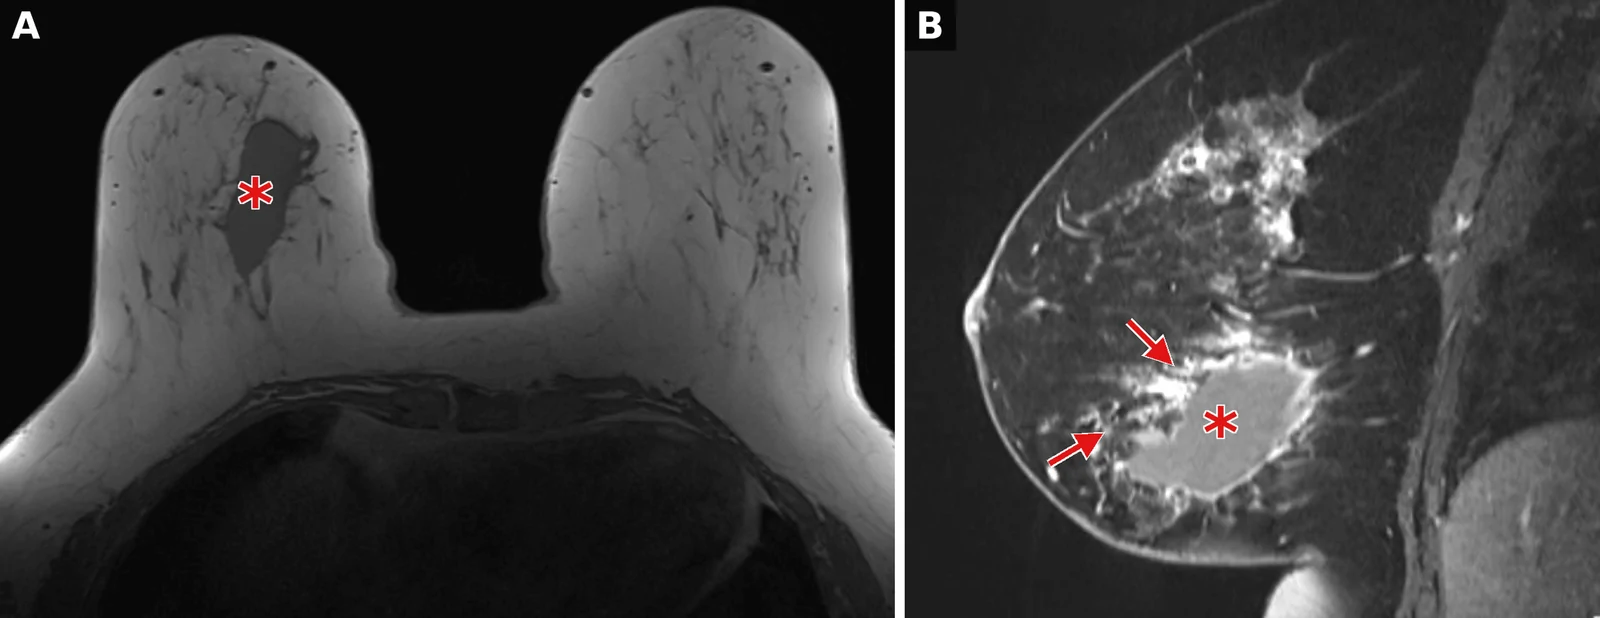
Postoperative breast MRI after wide local excision. (A) Axial T1-weighted image without fat suppression demonstrates a postoperative seroma in the right breast (asterisk). (B) Sagittal postcontrast T1-weighted fat-suppressed image of the right breast demonstrates the seroma (asterisk) with peripheral nodular enhancement along its anterior margin at the 5 o'clock position (red arrows), measuring 29x31x16 mm, consistent with residual tumor.
